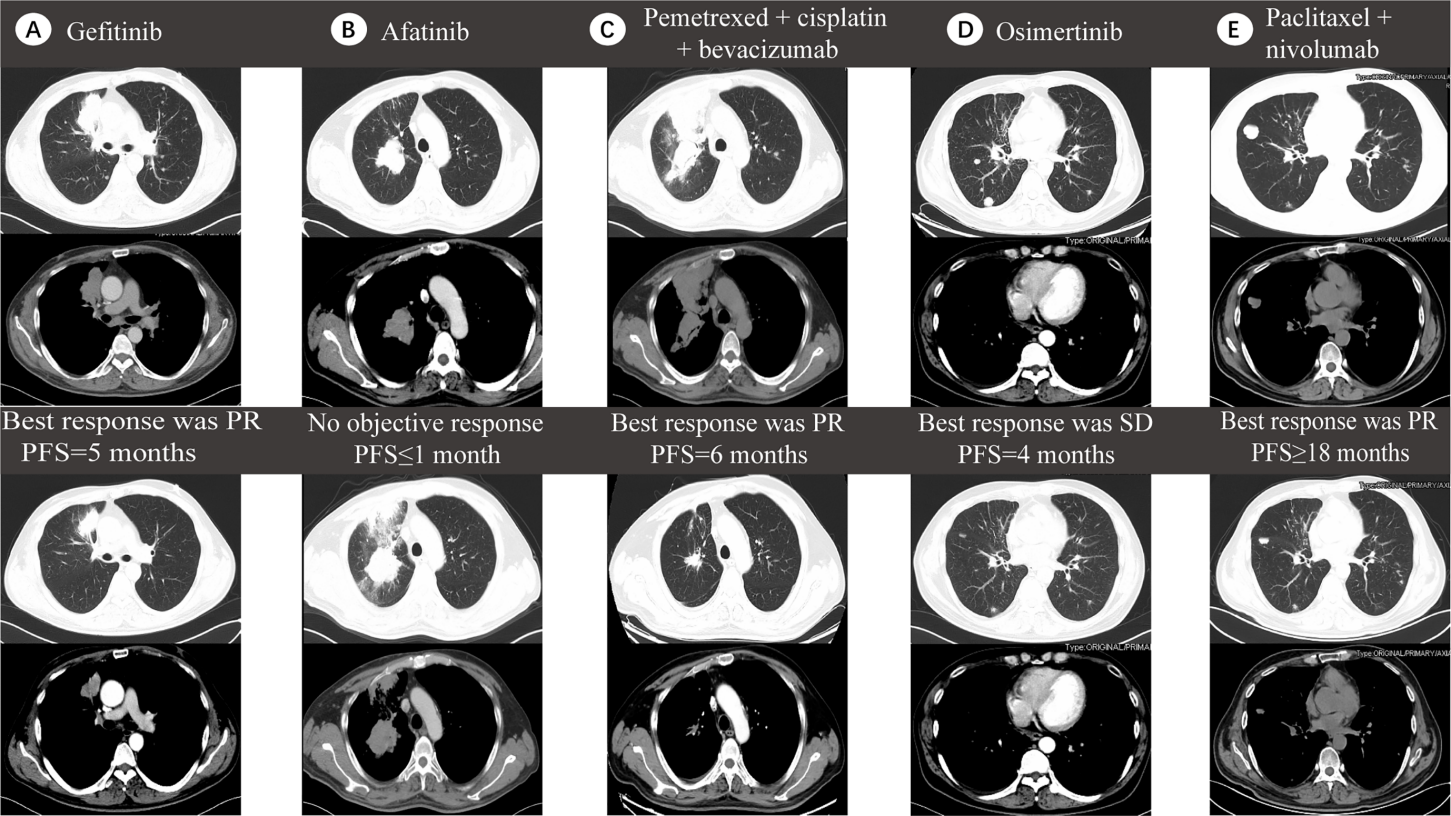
Five lines of treatment for case 2. (A) CT scan before (top) and after (bottom) receiving gefitinib, which produced a partial response (PR) in the right lung with 5 months of progression-free survival (PFS). (B) CT scan before (top) and after (bottom) receiving afatinib, which showed no objective response with less than one month of PFS. (C) CT scan before (top) and after (bottom) receiving pemetrexed, cisplatin and bevacizumab which brought a PR effect with 6 months of PFS. (D) CT scan before (top) and after (bottom) receiving Osimertinib, which induced no objective response with four months of PFS. (E) CT scan before (top) and after (bottom) receiving paclitaxel (albumin-bound) plus nivolumab which induced a PR effect with more than 18 months of PFS.
